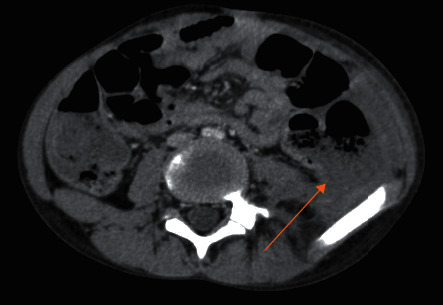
Abdominal CT: solution of continuity of the colonic wall with collection of neighbors (arrow).
